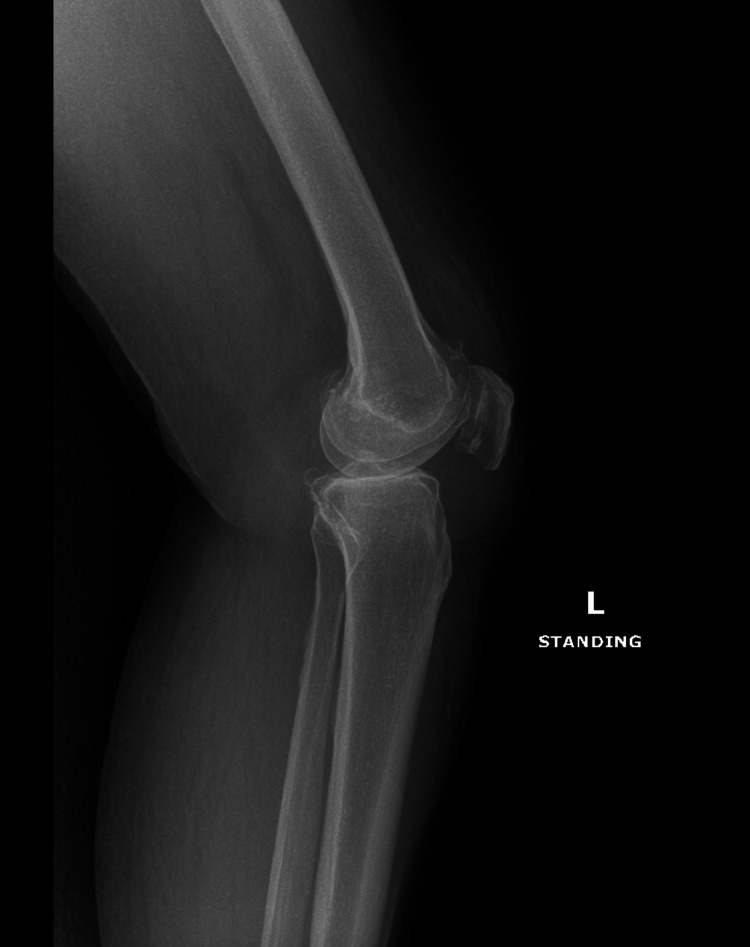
X-ray demonstrating a lateral view of the patients left knee.
